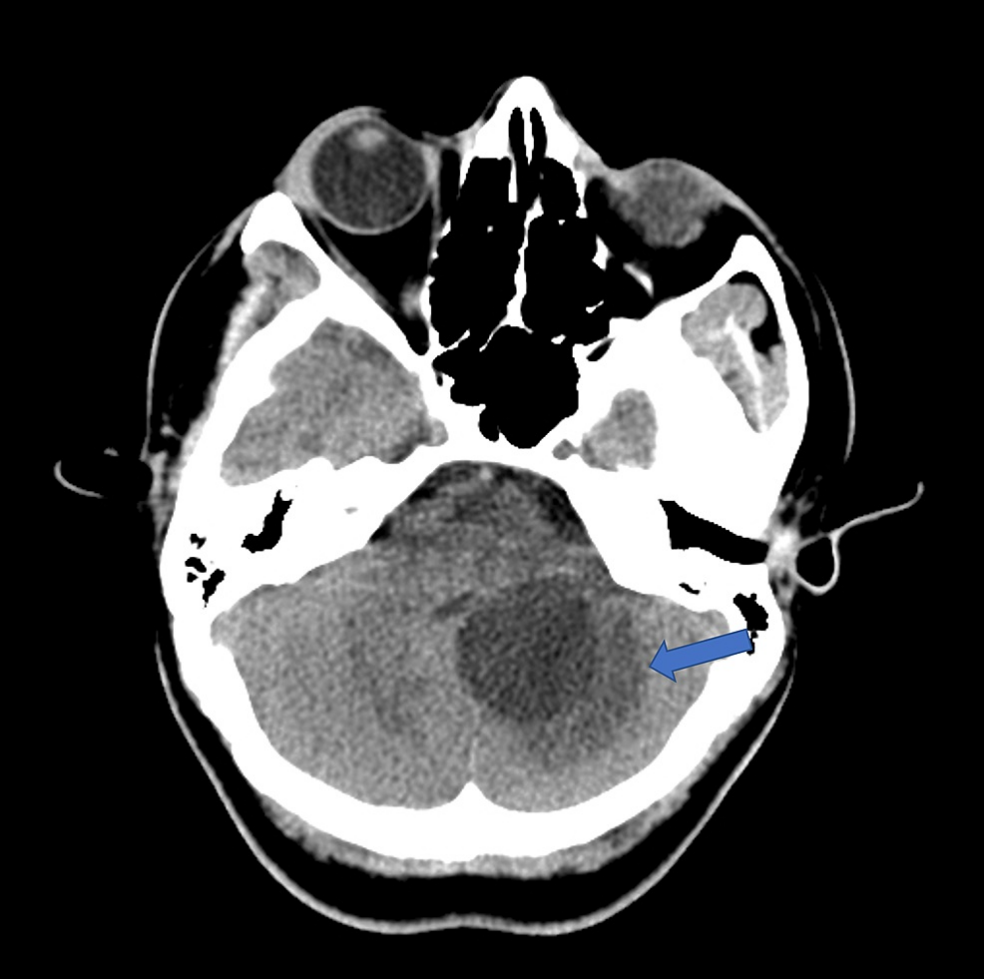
Axial CT without contrast Three cm cystic lesion in the posterior fossa, centered in the left medial cerebellar hemisphere with mass effect on the fourth ventricle and surrounding edema (blue arrow)
